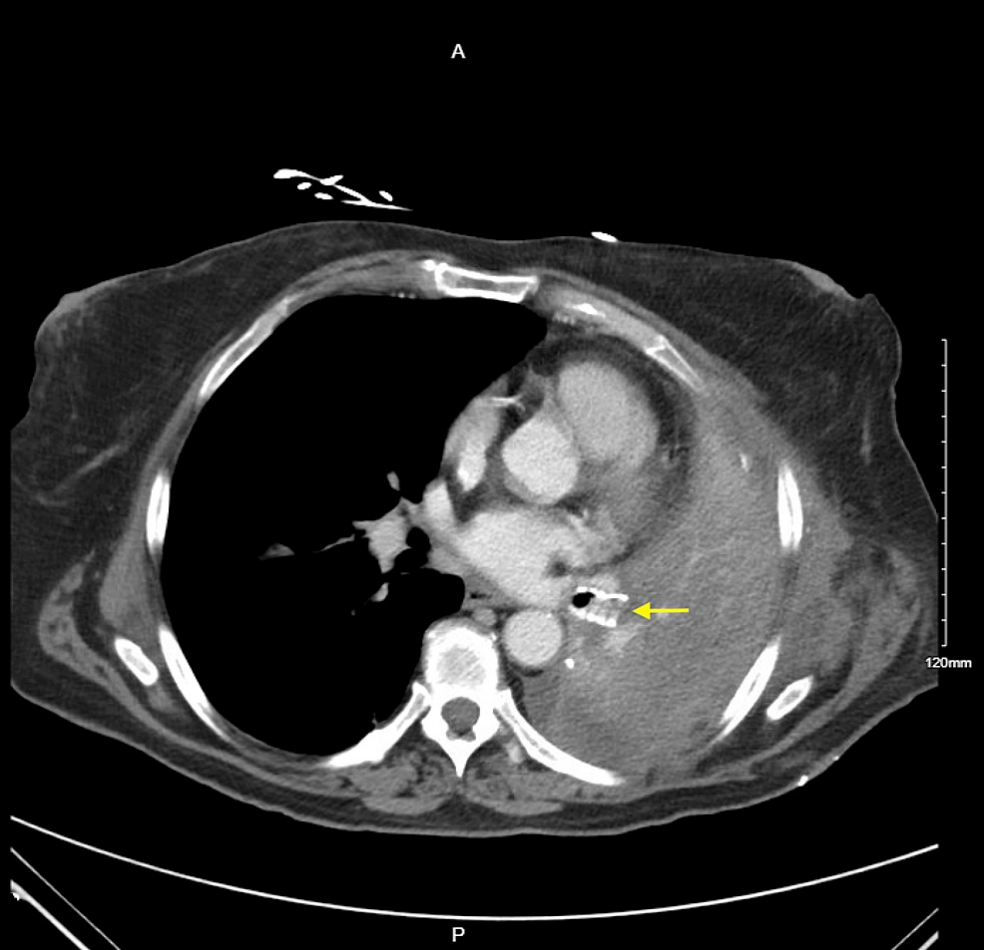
Postoperative CT chest showing the bronchial stent in place (yellow arrow).
